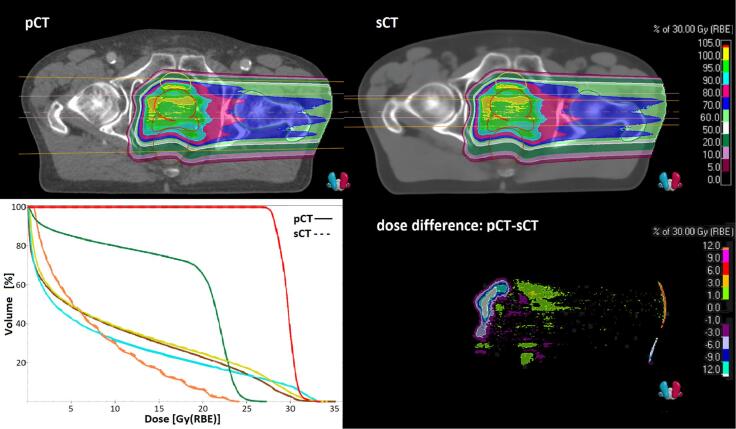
Representation of one of the proton fields (gantry 90°) that compose the proton plan for patient 2. Top row shows the dose contribution for the field for planning CT (pCT) (left) and synthetic CT (sCT) (right). The dose difference (pCT-sCT) (bottom row, right) displays the actual dose difference in percent. There is a slightly higher dose for the pCT before the target, and a lower dose behind. DVH (bottom row, left) shows the pCT dose (line) and the sCT (dotted line).
